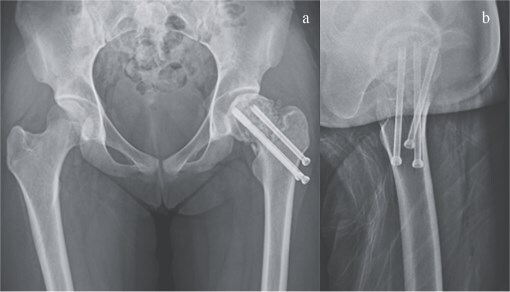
Plain radiograph after second surgery. (a) A-P view and (b) lateral view.
